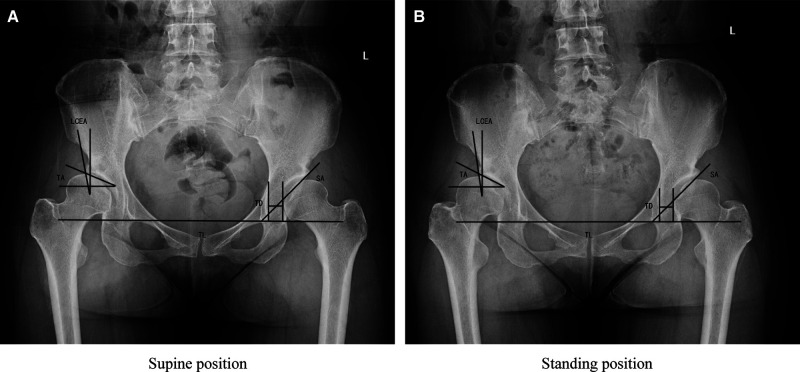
Supine (A) and standing (B) AP pelvic radiograph are shown. TL is the teardrop line; LCEA and TA are the lateral center edge angle and Tönnis angle, TD and SA are the teardrop distance and sharp angle. TD in the standing position was larger than that in the supine position.
